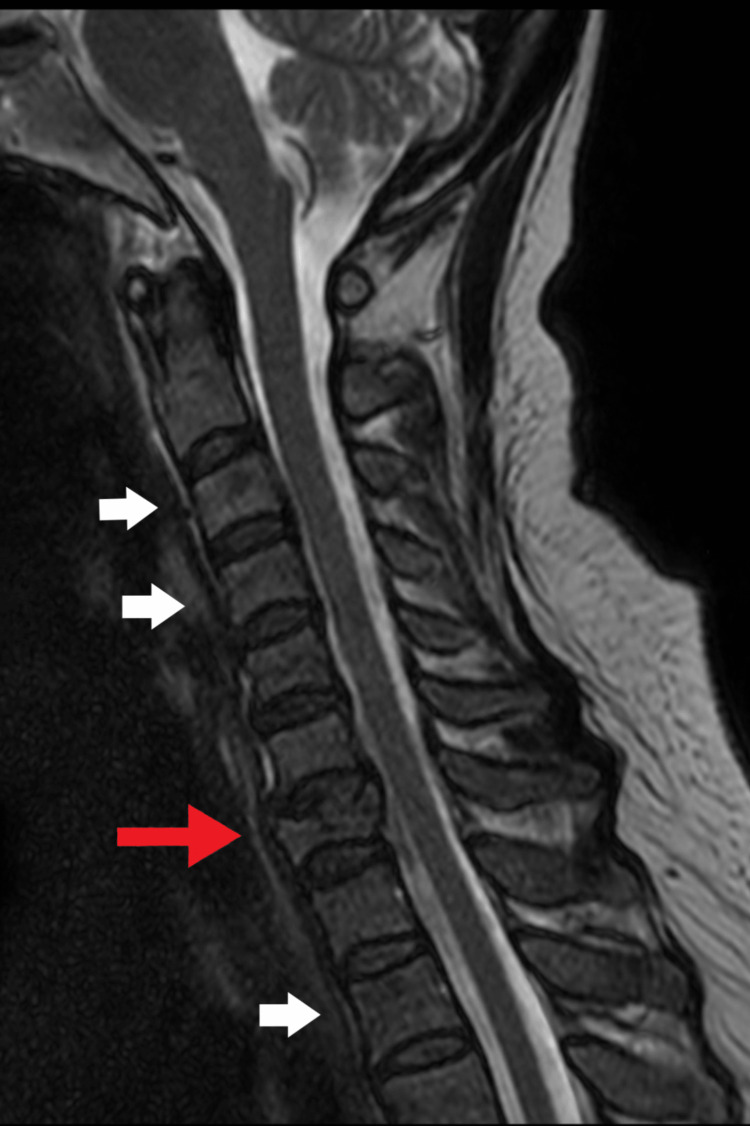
Sagittal view of the cervical MRI. Multiple patchy heterogeneous T1W hypointense T2W hyperintense marrow signals are visible at the cervical spine, including the upper thoracic spine and clivus. Partial collapse of the C7 vertebral body with mild retropulsion is noted. Possibility of bone metastasis/infiltrative marrow disease with pathological fracture of C7 was considered.
